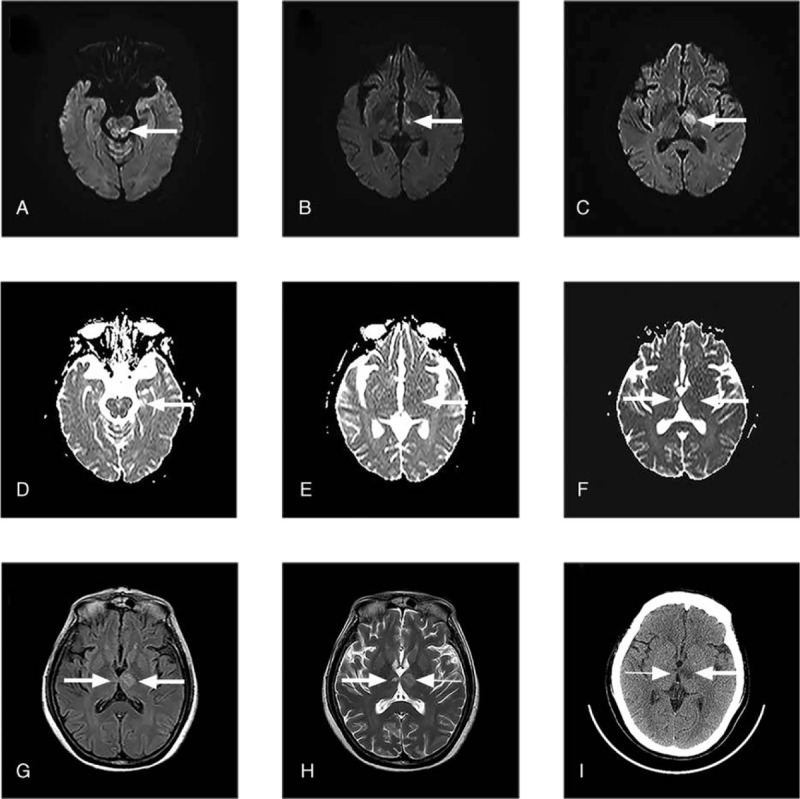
(A–C) Diffusion weighted axial images showing hyperintense signal in mesencephalic and left paramedian thalamic lesions (arrows). (D–F) Apparent diffusion coefficient images demonstrating decreased (or equal) signal in the same lesions and hyperintense signal in right paramedian thalamic lesion (arrows). (G, H) Axial FLAIR and T2 sequence images demonstrating hyperintense signal in the bilateral paramedian thalamic lesions (arrows). (I) Cerebral computed tomography showing bilateral paramedian thalamic lesions (arrows).
